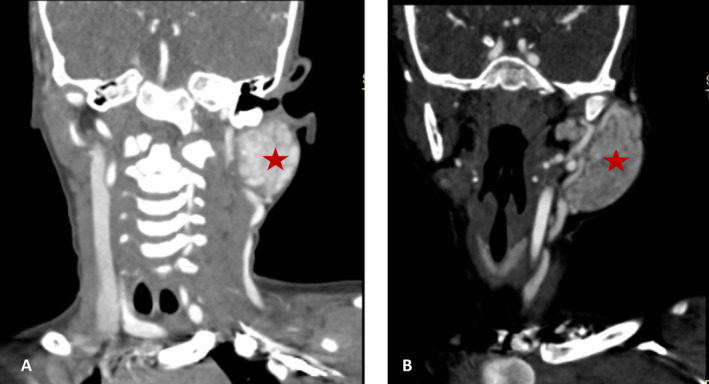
(A) and (B) are contrasted head and neck CT coronal reformats with the mass showing avid enhancement in the arterial phase, with retention of contrast in the venous phase, respectively. This makes this mass (red star) an arteriovenous malformation.
